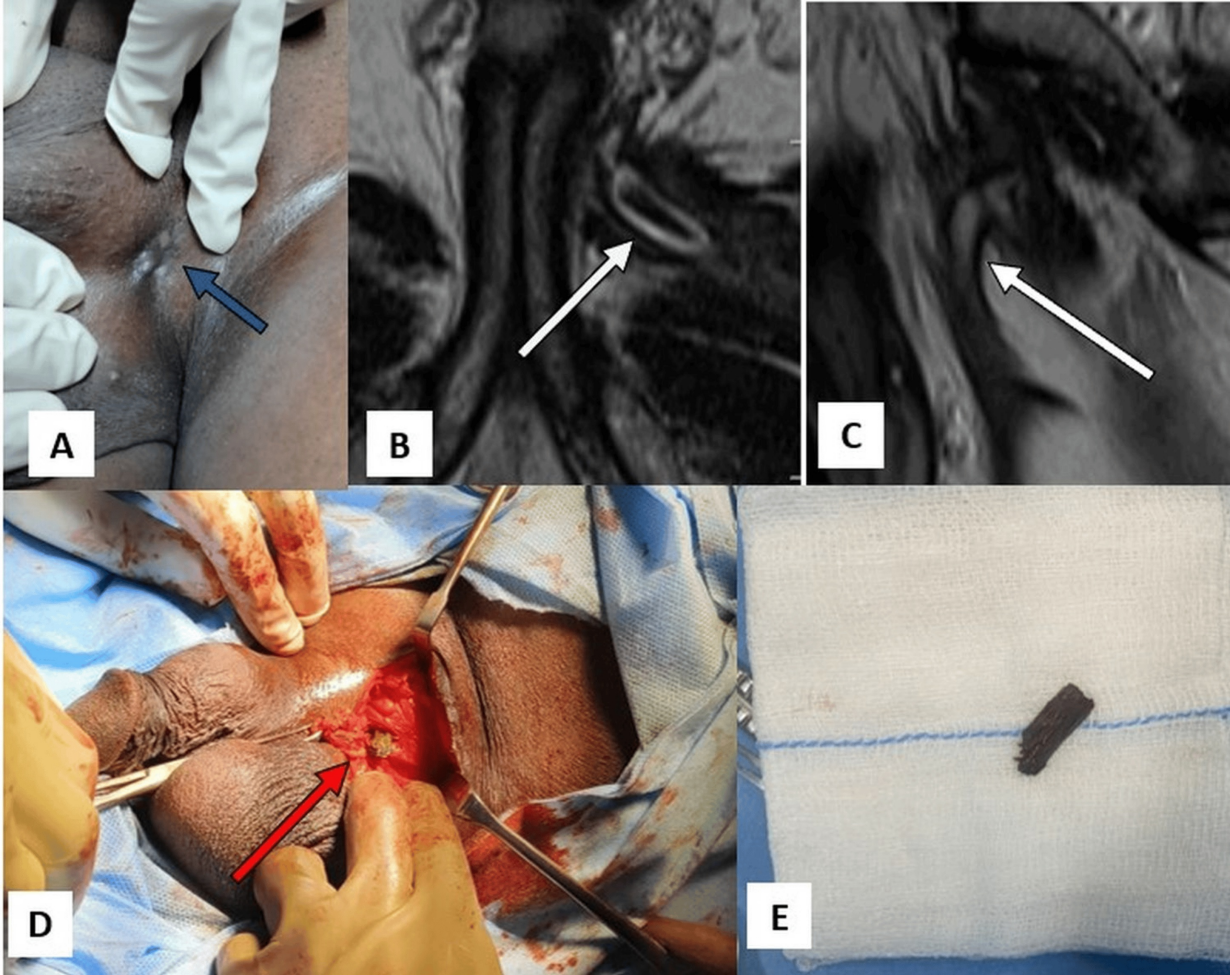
Imaging and surgical management of penetrating traumatic urological foreign body (Case 2). (A) Clinical photograph showing a discharging sinus at the left scrotal root (blue arrow). (B) Axial T1-weighted MRI revealing a sinus tract extending into the adductor region (white arrow). (C) Sagittal T1-weighted MRI showing the trajectory of the tract containing the foreign body (white arrow). (D) Intraoperative image demonstrating retrieval of a wooden splinter from the tract (red arrow). (E) Extracted organic foreign body placed on a surgical gauze.
